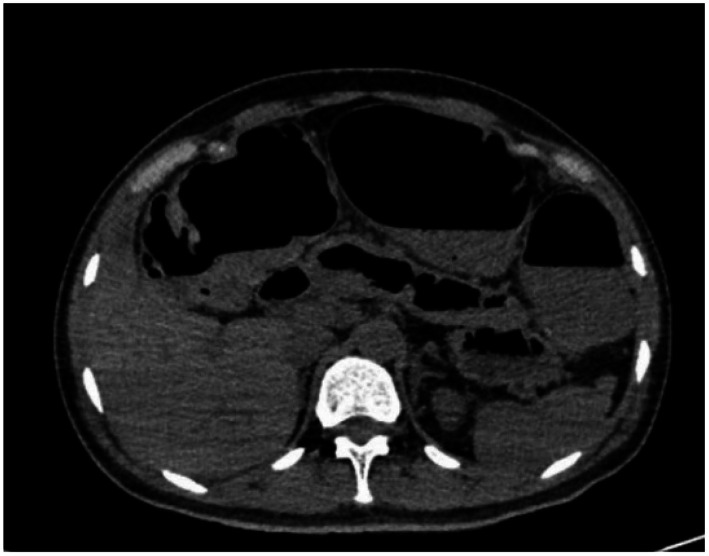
Chest, abdomen, and pelvis CT: multiple small bowel dilations with pneumatosis and multiple fluid levels, consistent with changes in intestinal obstruction.
